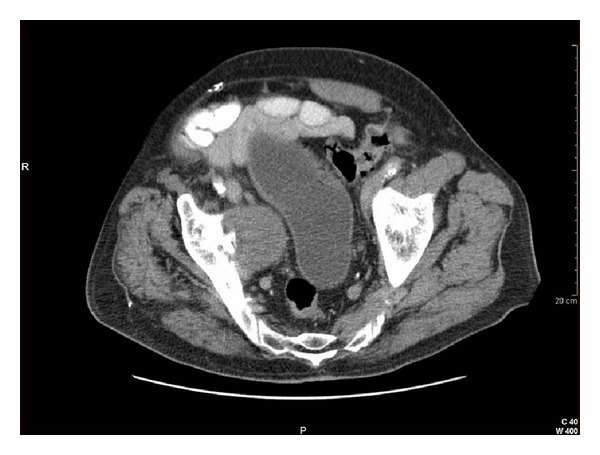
CT scan of pelvis revealing large mass to be extrinsic to bladder wall.
